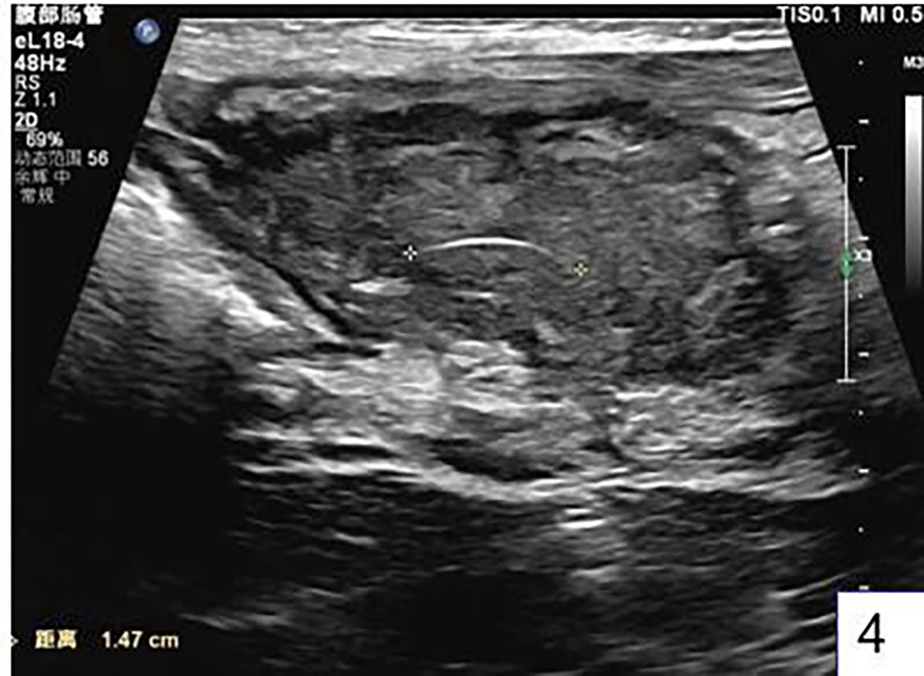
Color ultrasound examination found that there was pus in the mass, and a curved foreign body about 1.6 cm in length was found in the pus cavity.
